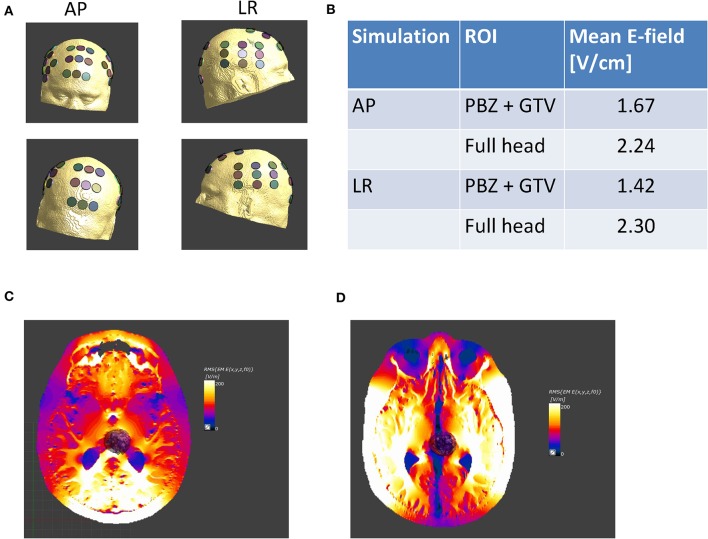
Tumor-treating fields intensity distribution. (A) Following model creation, virtual transducer arrays were placed on the patient model. The arrays were placed to match the array layout placed on the patient, as recorded in the clinical records, anterior-posterior (AP) and left-right (LR); (B) average field intensities within the GTV, comprising a region of enhancing tumor visible on the T1 contrast MRI at baseline and a PBZ 3 mm wide surrounding the tumor, were calculated. The table summarizes the average field intensity delivered by each pair of arrays to a tumor bed comprising the joint volume of the GTV and PBZ, as well as to the entire head. The table clearly shows that within the tumor bed, field intensities exceed the therapeutic threshold of 1 V/cm; electric field distribution shown for the AP (C) and LR (D) TTFields array configuration.
